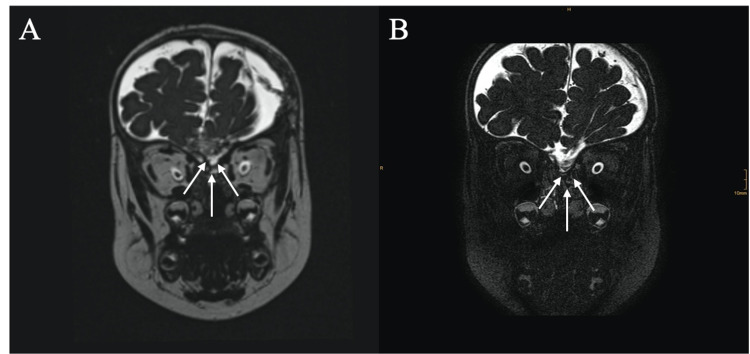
(A) A postoperative MRI control with a coronal T2-weighted sequence displays successful removal of the meningoencephalocele from the left ethmoidal sinus (white arrows). (B) At the six-month follow-up, the MRI control with coronal T2-weighted sequences shows complete closure of the skull base defect, thus a satisfactory result of the previously performed combined surgical approach for the treatment of the meningoencephalocele (white arrows).
